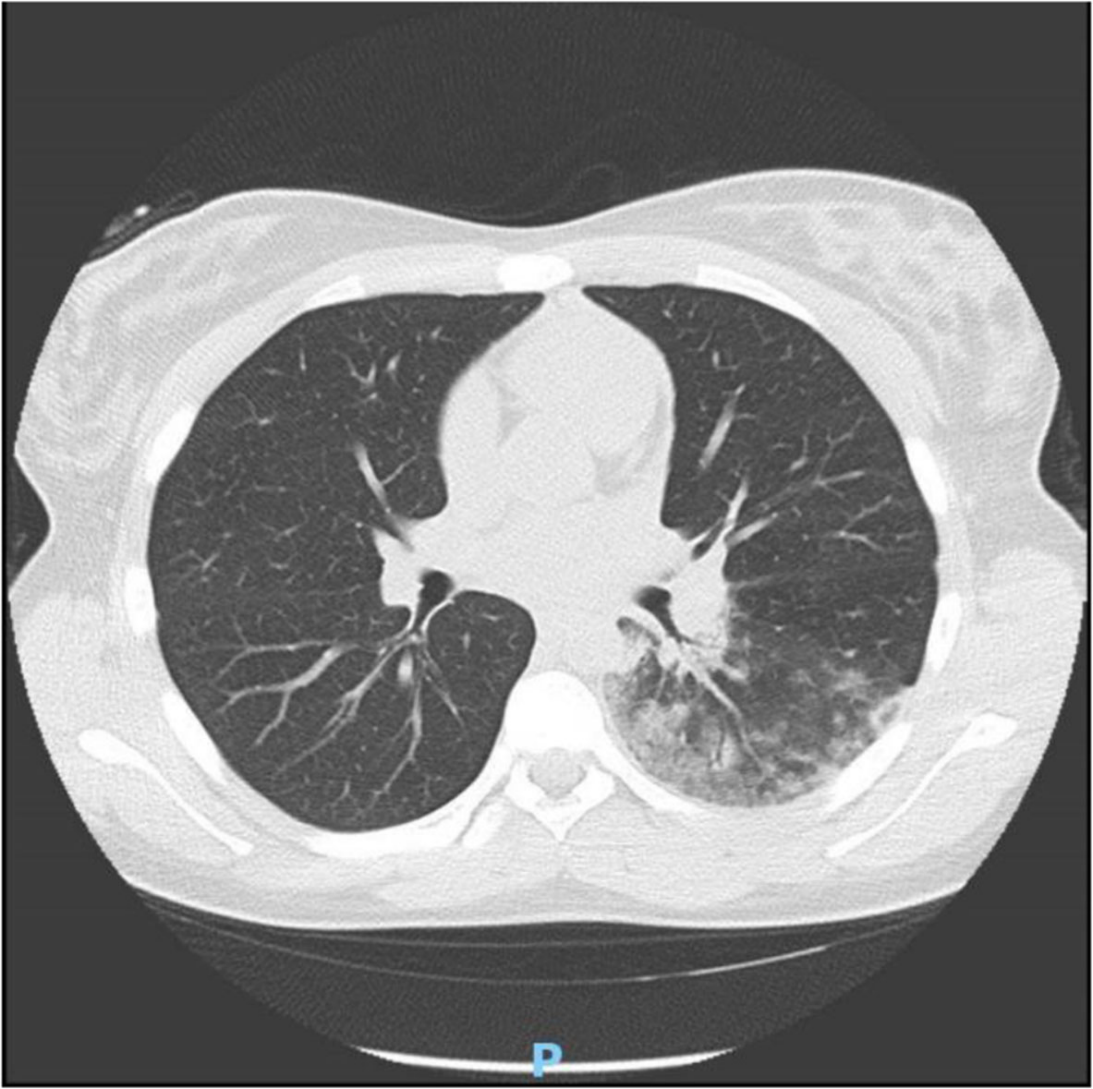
Patient’s lung CT: Multiple round and oval ground-glass opacities in the left lung
